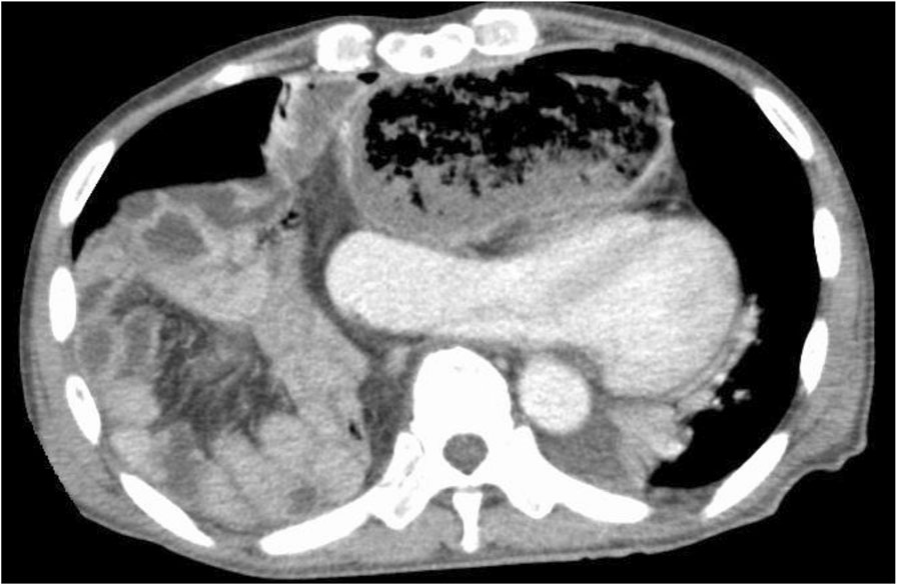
Computed tomography findings of case 1. The axial image demonstrated that the intestine had been drawn into both thoracic cavities. The intestine pressed the gastric conduit, leading to obstruction and dilation. This dilated gastric conduit severely pressed the heart
